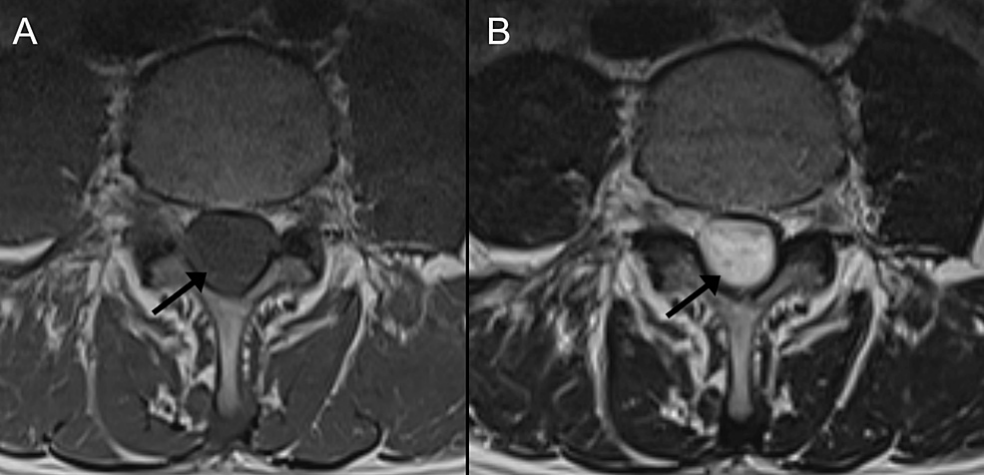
Lumbar spine magnetic resonance imaging, axial views at the level of L4. A mass occupies the entire spinal canal (arrows) which is isointense on the T1-weighted sequence (A), and slightly hyperintense on the T2-weighted sequence (B).
